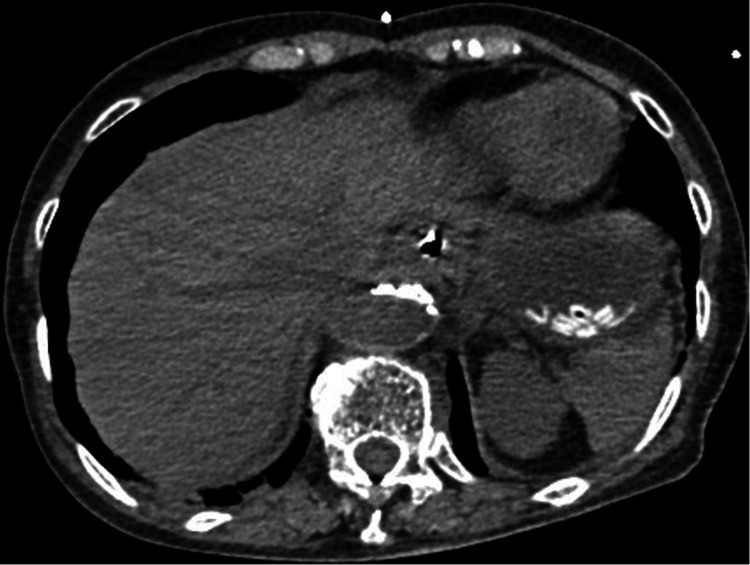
Abdominal CT scan showing multiple high-density materials in the stomach, consistent with ingested tablets.
